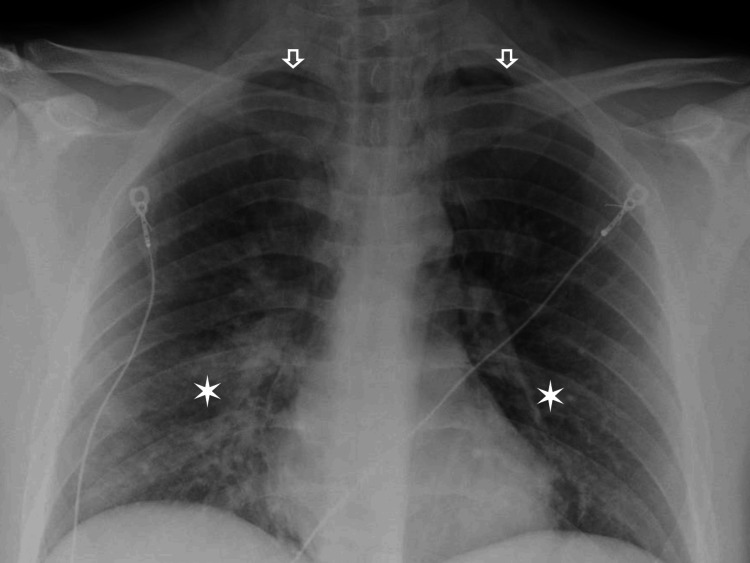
Upright Chest Xray The star notes the basilar opacities. The arrow indicates trace bilateral pneumothoraces
